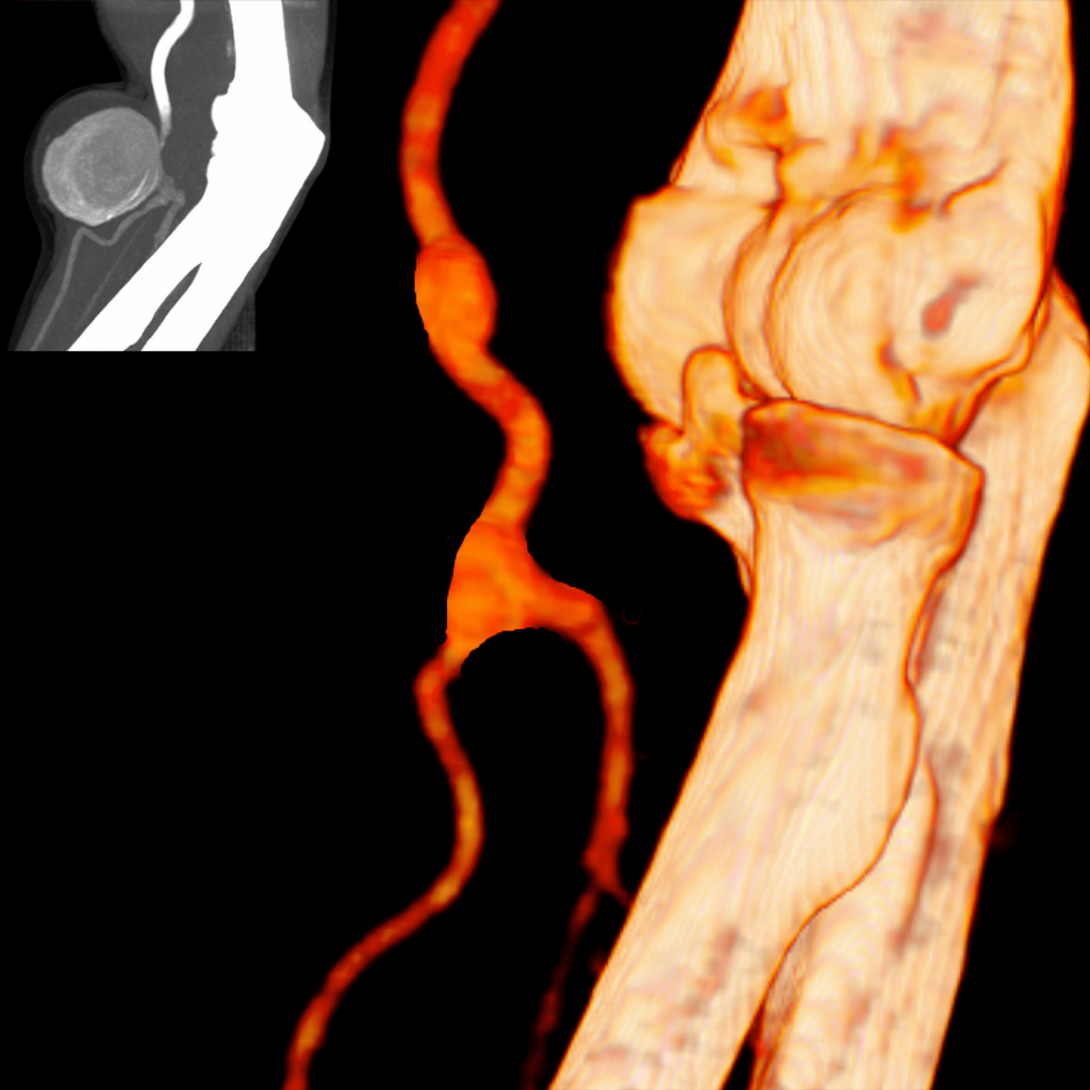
12-month follow-up computed tomography angiography 3D reconstruction, showing the arterial reconstruction after surgical excision of the brachial artery aneurysm
